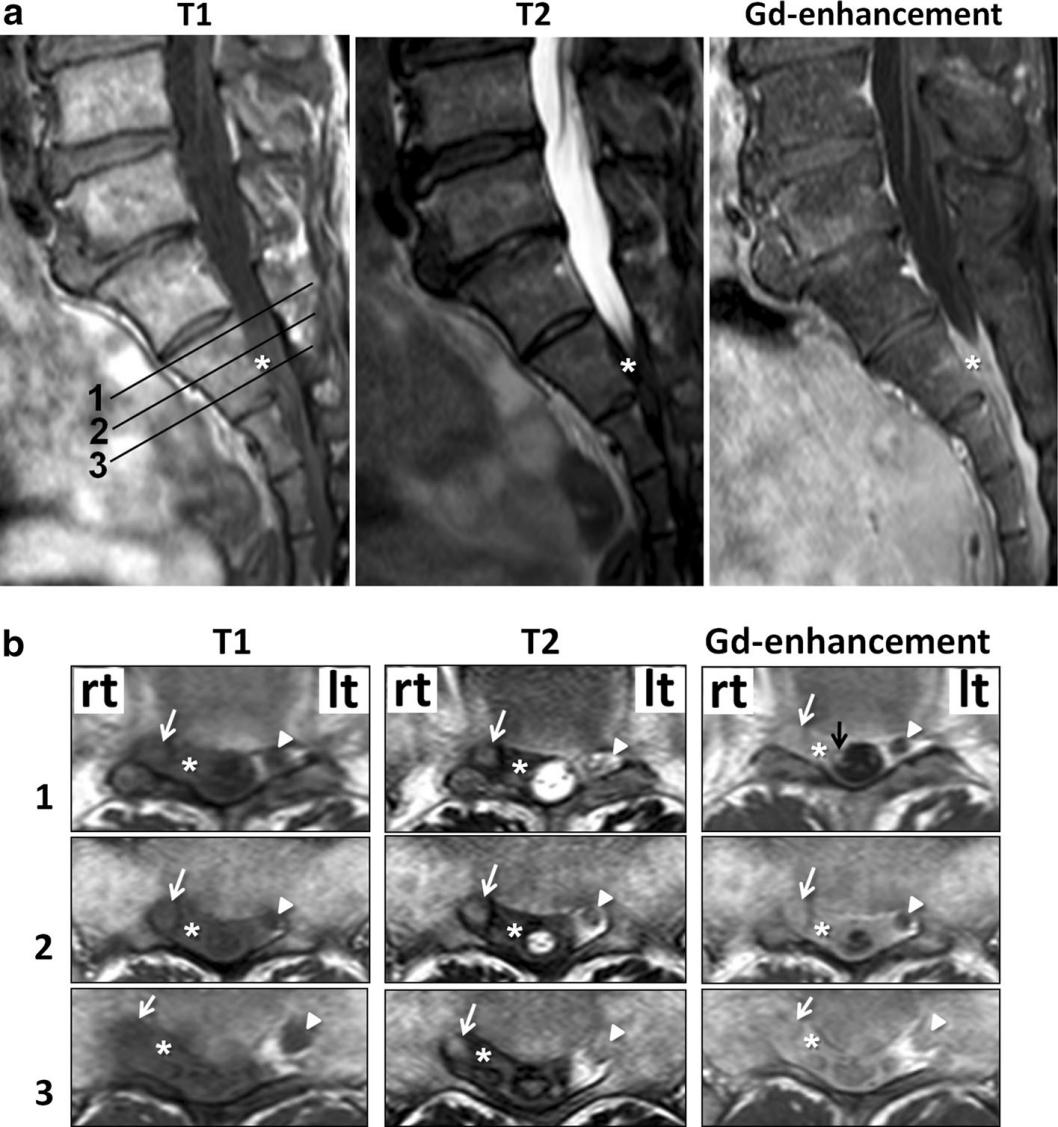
The *numbers* in the sagittal T1-weighted MRI (a) indicate the slice levels in the axial images (b). *Asterisks* a space occupying lesion (SOL). *White arrows* (*right*) and *arrow heads* (*left*), S2 nerve root. *Black arrow* slightly enhanced cauda equina
